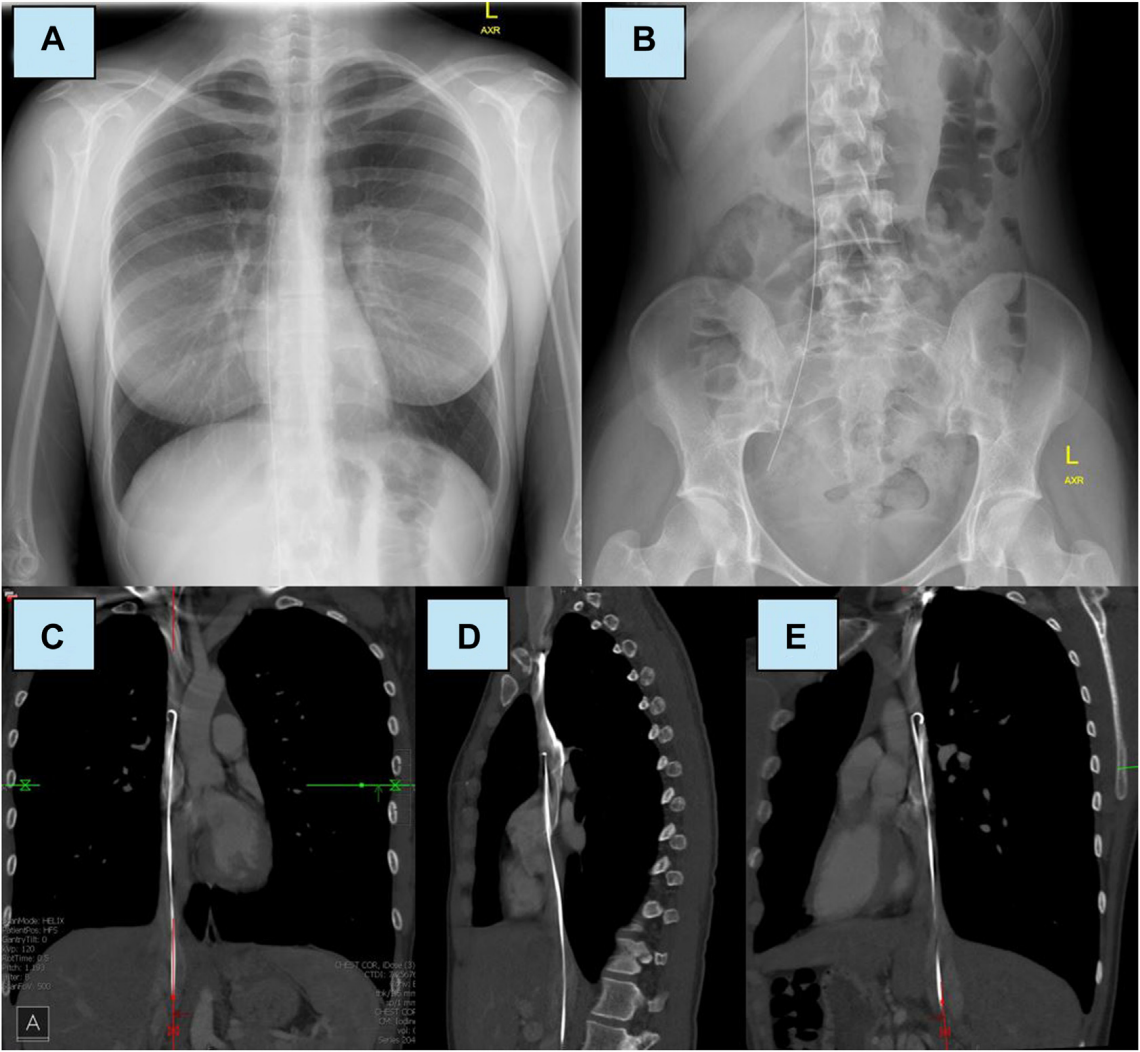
A: Chest radiograph on presentation reveals the retained J-wire extending into the superior vena cava along the right edge of the vertebral column. B: Abdominal radiograph shows the termination of the retained wire in the femoral vein. C: Coronal computed tomography (CT) scan revealing the thoracic course of the retained J-tipped wire. Multiple sections of wire are free-floating within the vein. D: Sagittal-plane CT scan showing the same. E: Multiplanar reconstruction CT scan showing the same from a posterolateral oblique plane.
